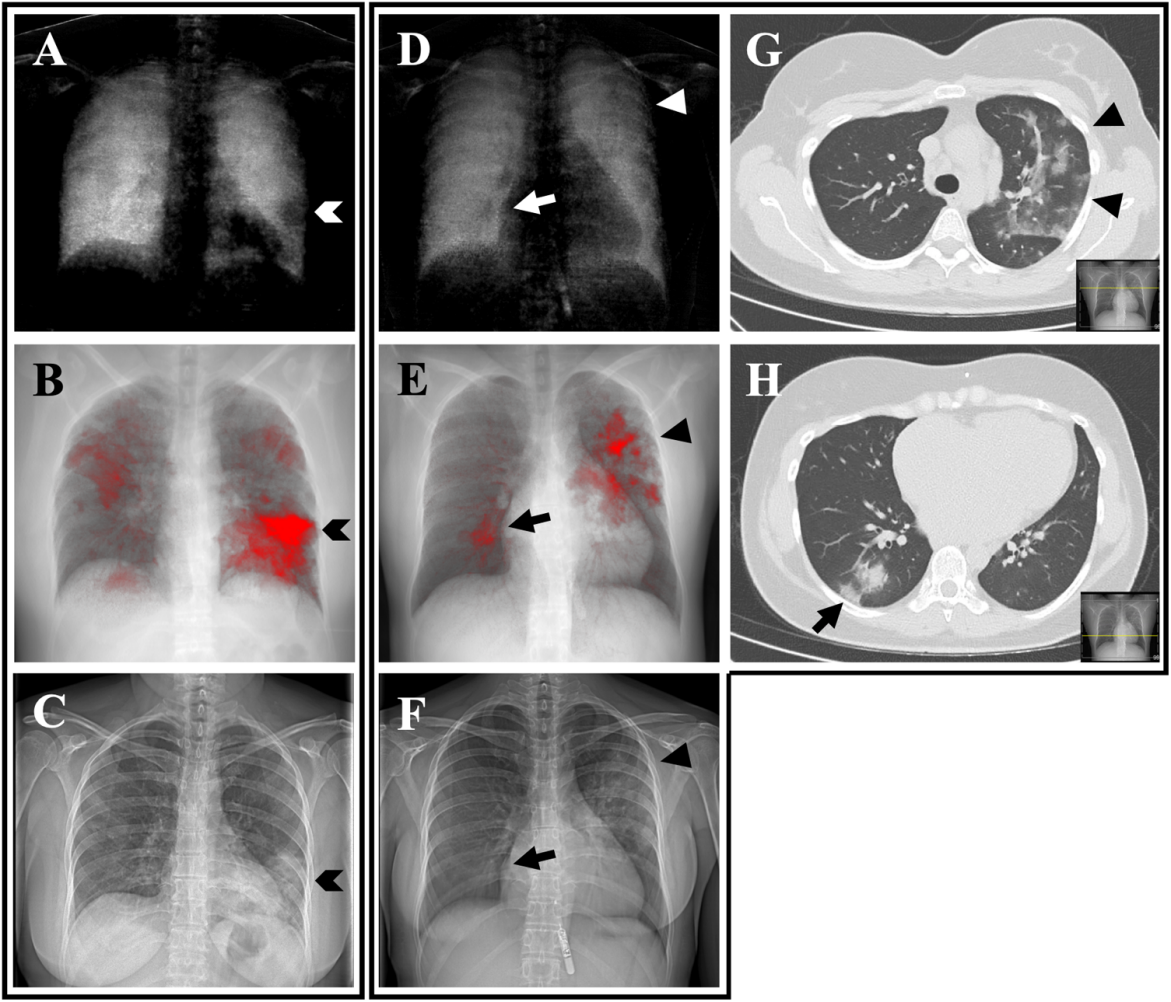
Dark-field radiographs (A,D), CT-based projections (B,E), conventional radiographs (C,F) and axial reformations (G,H) of a CT scan from a 47-year-old woman (A–D) and a 36-year-old woman (D–F, G,H). In the participant on the left, the focal signal loss in the periphery of the left lower zone (A, arrowhead) corresponds well to the red area in the CT-based projection (B, arrowhead) and the opacity in the conventional radiograph (C, arrowhead). The same applies for the second patient (D–F) with focal signal loss in the right lower zone (arrow) and the left upper zone (triangle). The corresponding axial CT reformations (G,H) reveal opacities in the respective regions.
